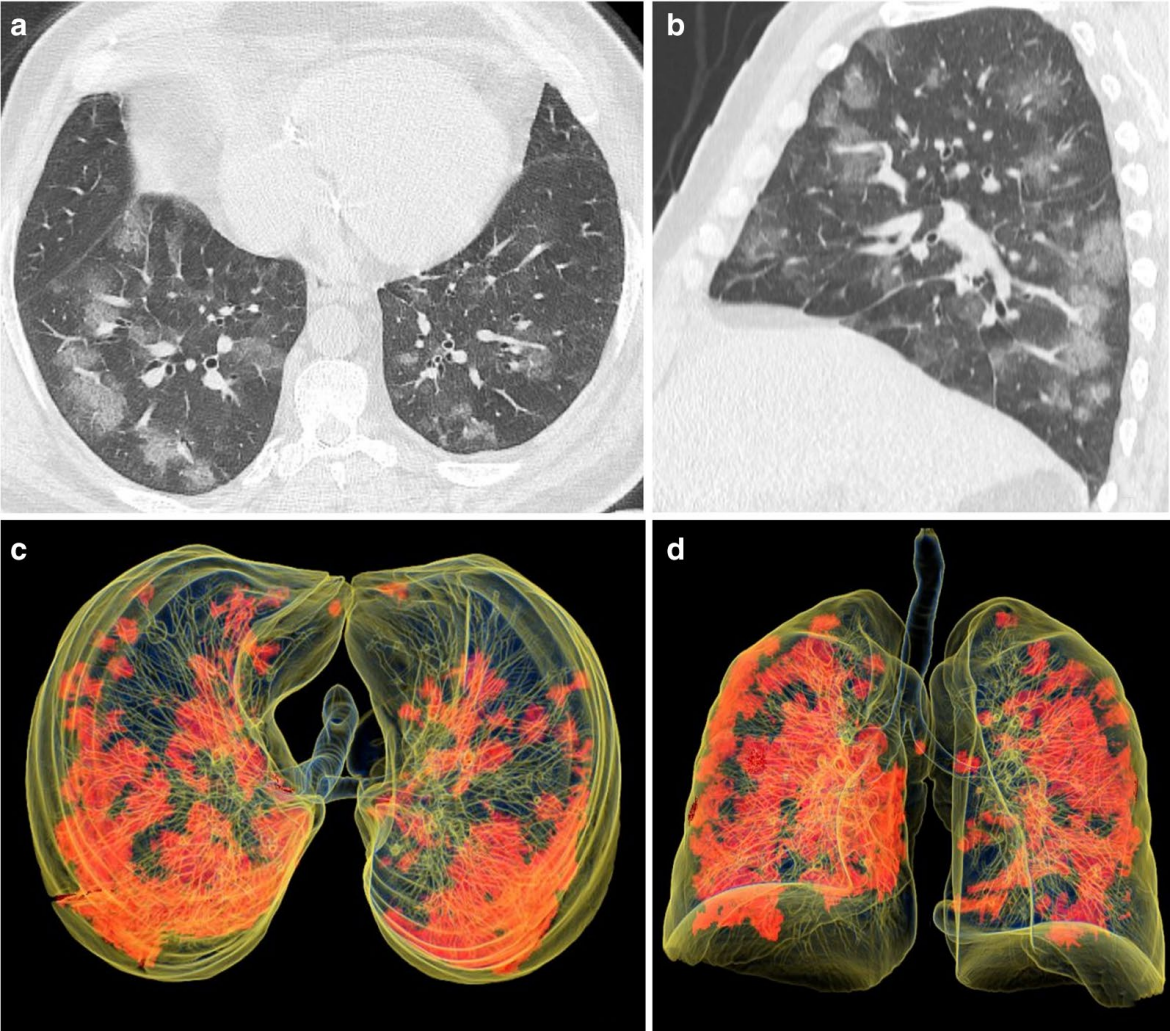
Typical CT imaging features for COVID-19. a, b A 54-year-old man with positive RT-PCR test, axial and sagittal reformatted CT images shows multifocal rounded GGO with predominant peripheral distribution. c, d Three-dimensional semitransparent volume-rendered reconstructions show the peripheral and basal distribution of the ground-glass opacities across both lungs
